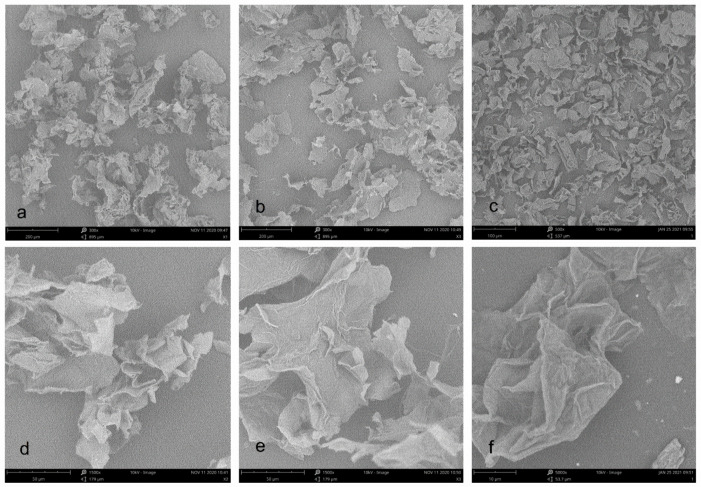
SEM images of cellulose from pomelo fruitlets: (a) CAA at 300× magnification; (b) CA at 300× magnification; (c) CUA at 500× magnification; (d) CAA at 1500× magnification; (e) CA at 1500× magnification; (f) CUA at 5000× magnification. CAA, CA, and CUA are cellulose prepared by acidic-alkaline hydrogen peroxide hydrolysis, alkaline hydrogen peroxide hydrolysis, and ultrasonic assisted alkaline hydrogen peroxide hydrolysis, respectively.
